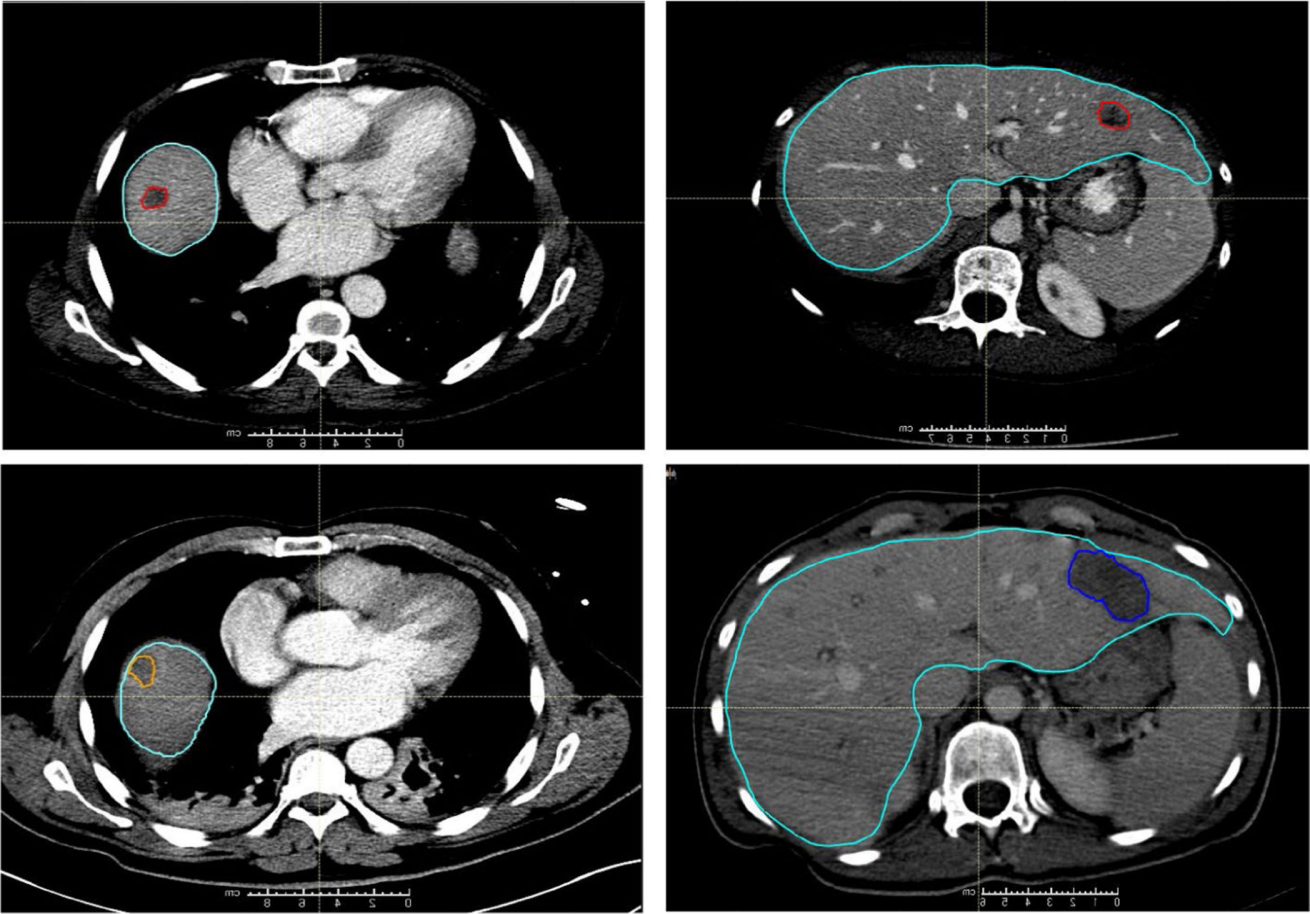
Computed tomography (CT) scans from two patients are shown, one in the left column and one in the right. (Top Row) Contouring of colorectal liver metastasis from diagnostic contrast-enhanced CT (red) and the liver contour (teal). (Bottom Row) Contouring of the ablation region (orange on the left and dark blue on the right) on the CT scan obtained immediately following the ablation
